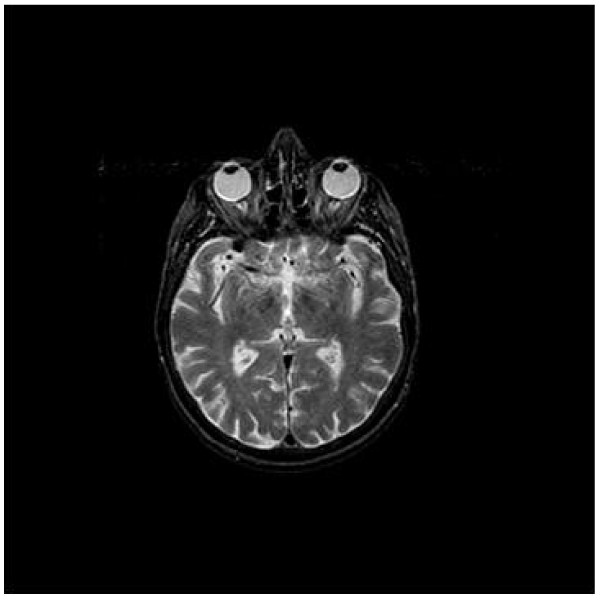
Brain MR image. Figure displays a normal brain MR image. The image is downloaded from the Whole Brain Atlas, Harvard University.
